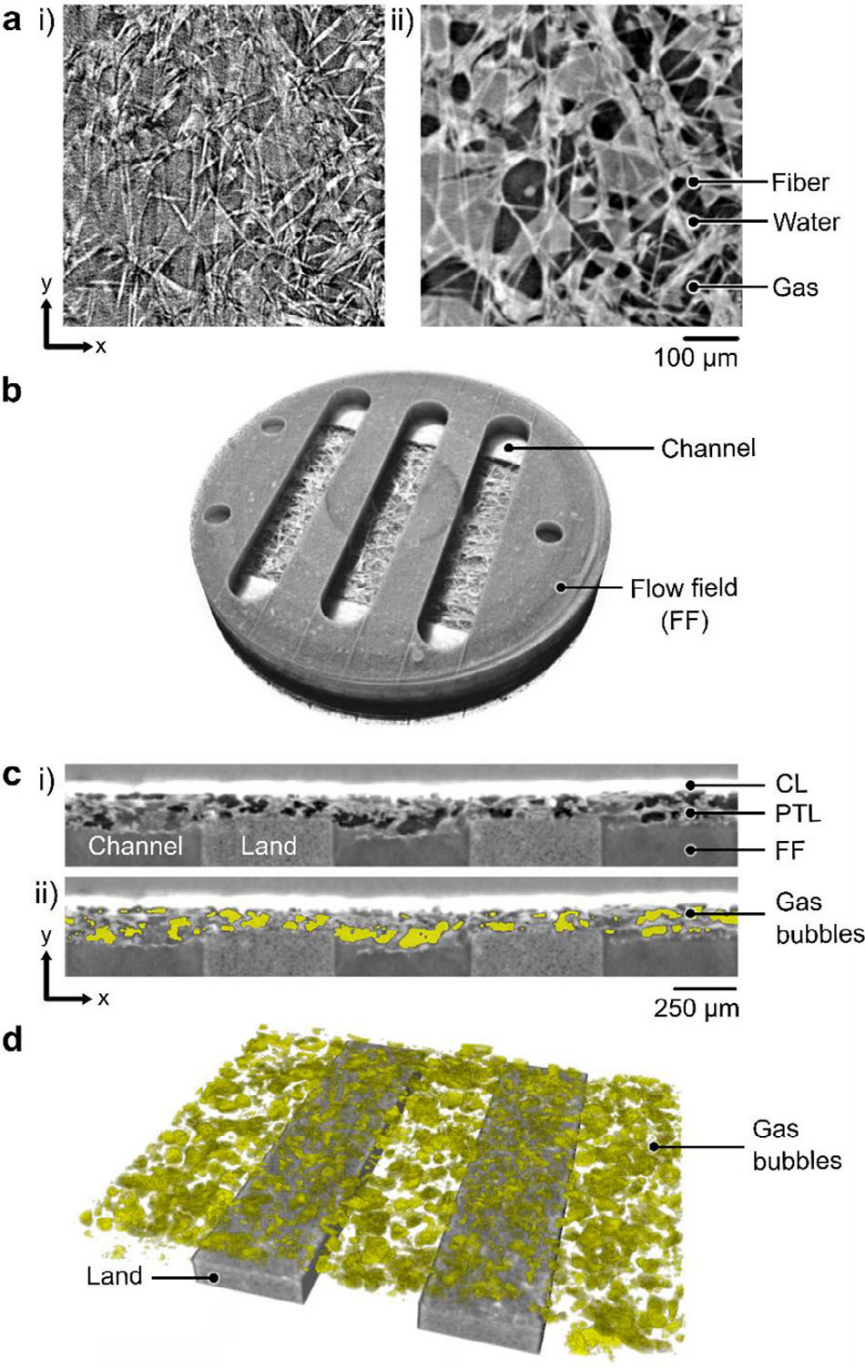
Image processing and segmentation of 3‐D gas distributions: a) A reconstructed slice (i) without and (ii) with phase retrieval. Phase retrieval reconstruction enhanced contrast between phases of fiber, water, and gas. b) 3‐D rendering of the single cell PEM electrolyzer housed between graphite FFs with three 0.5 mm wide channels. c) Gray scale threshold segmentation of gas bubbles in the cropped region of interest. The cropped gray scale image stack in (i) includes the CL, carbon PTL, and FF with three channels and two lands. The segmented gas distribution is overlaid in yellow onto the image stack in (ii). d) 3‐D volume rendering of segmented gas bubble distribution in the anode compartment overlaid onto anode FF with two 0.5 mm wide lands. Operando X‐ray CT successfully visualized pore‐scale gas bubbles.
